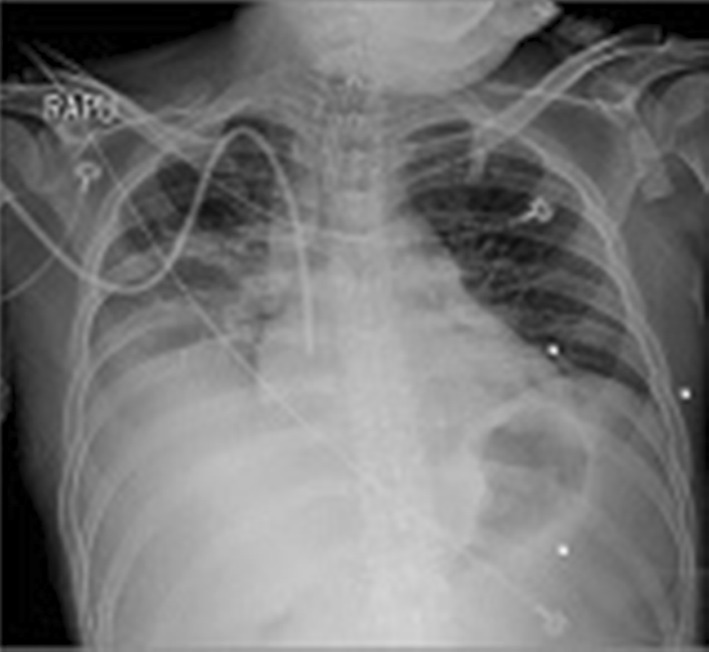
Chest X-ray showed infiltrations in right upper and lower lung fields
